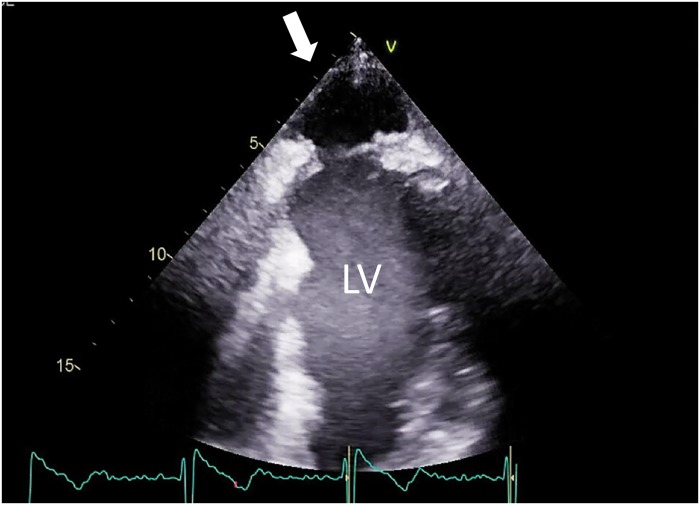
Transthoracic echocardiogram in a two-chamber view shows a 40 × 27-mm pseudoaneurysm (white arrow), connecting with the left ventricle around the apex of the heart. LV, left ventricle.
